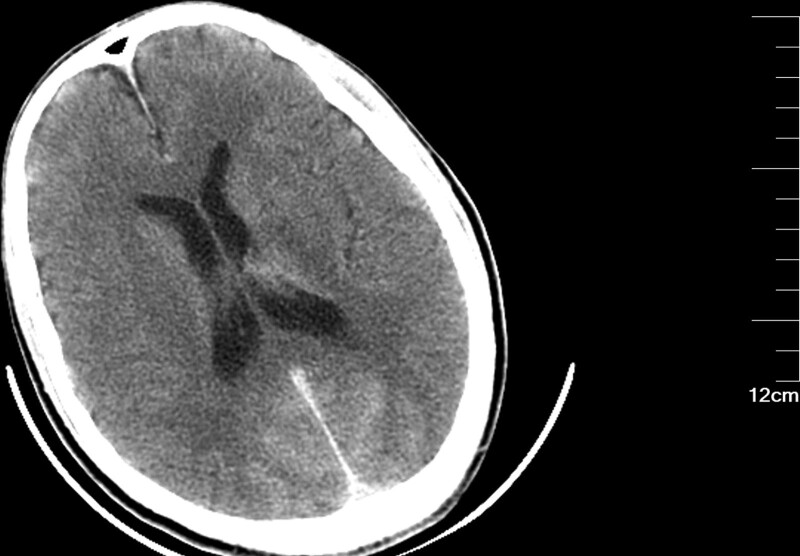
Imaging after cerebral angiography. The brain CT showed subarachnoid enhancement in the occipital region. CT = computed tomography.
